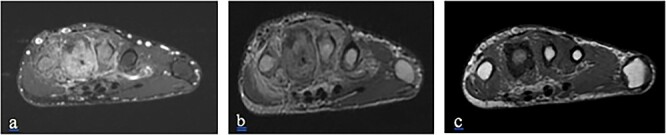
MRI of the left hand, an axial section, on T1weighted sequence (c) and T1with fat saturation (b) sequences, showing cortical thickening of the 3rd, 4th and 5th metatarsal, as a hypointense signal, enhanced after injection (a) with infiltration of the surrounding soft tissue.
